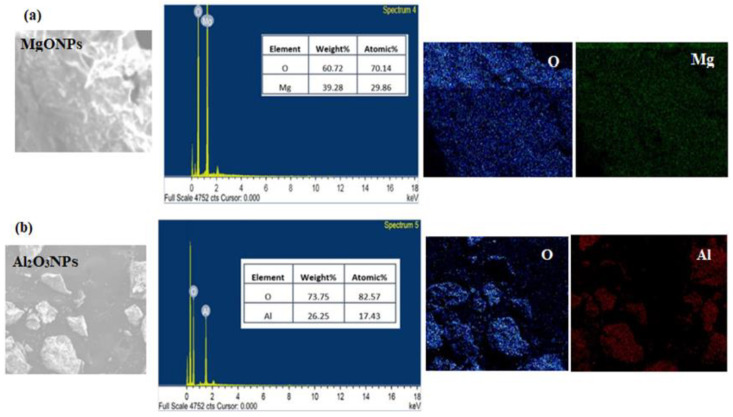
EDX analysis and elemental X-ray mapping of green synthesized (a) MgONPs and (b) Al_2_O_3_NPs using *Salvia officials* leaves and *Cuminum cyminum* seed extract, respectively.
